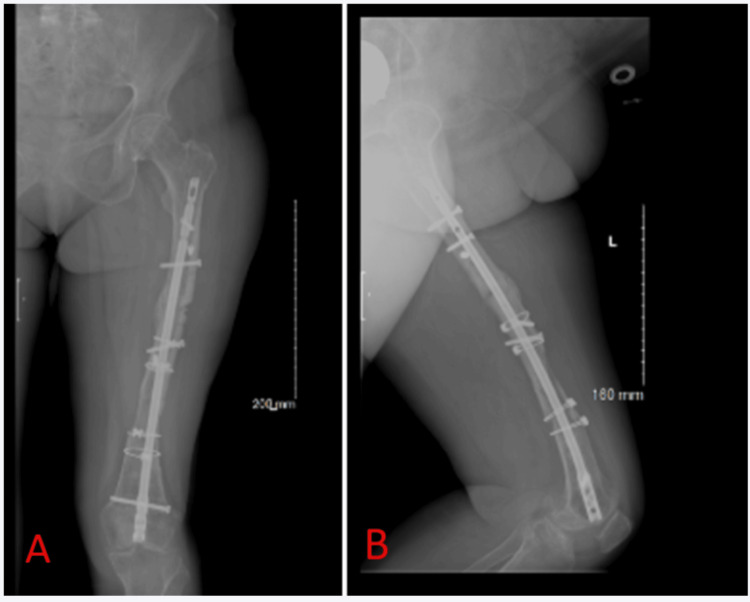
X-rays obtained 19 months postoperatively after our revision surgery A: Anteroposterior X-ray of the left femur; B: Lateral X-ray of the left femur
